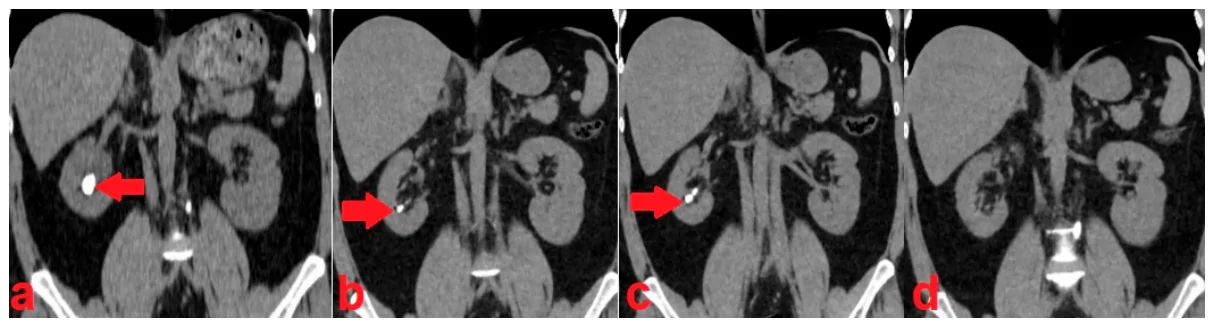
Serial radiological imaging of a patient managed with secondary percutaneous nephrolithotomy (repeat PCNL): (a) Preoperative non-contrast computed tomography (NCCT) demonstrating an isolated left lower-pole calculus measuring 20.0 mm in maximum diameter (arrow); (b) Baseline NCCT obtained at 3 months postoperatively (study time zero) showing a 6.0 mm residual stone fragment (arrow) establishing study eligibility; (c) Surveillance NCCT at 60 months postoperatively revealing interval fragment enlargement to 15.0 mm (arrow); and (d) Control NCCT confirming complete stone clearance following secondary PCNL at 63 months postoperatively (terminal stone burden: 0.0 mm^2^).
